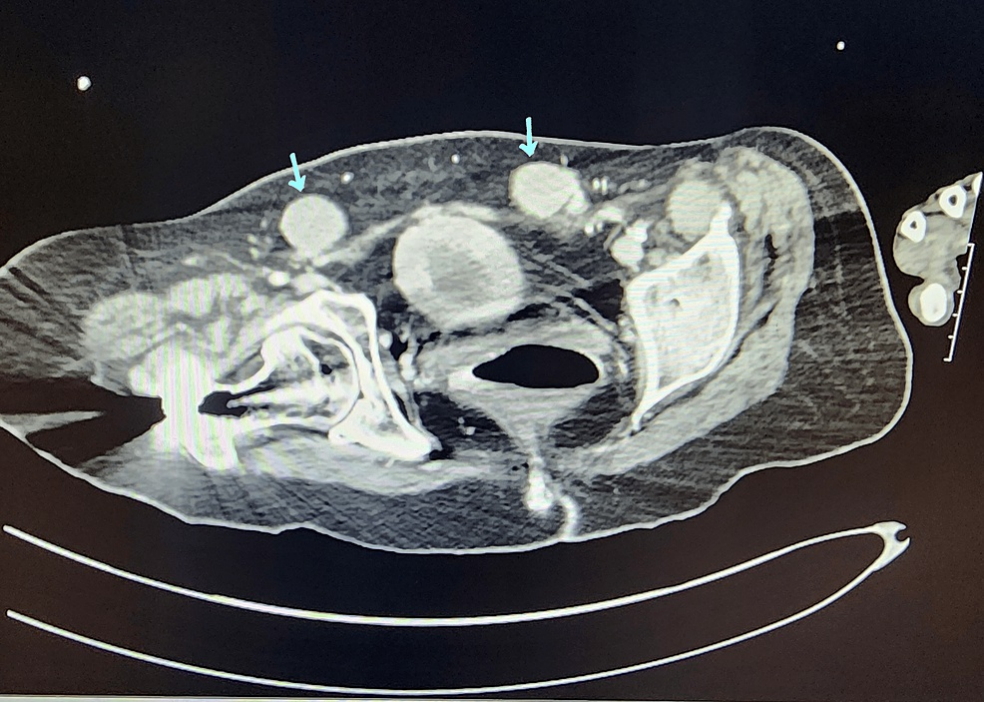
CT scan axial view. Blue arrows showing bilateral undescended testicles.
